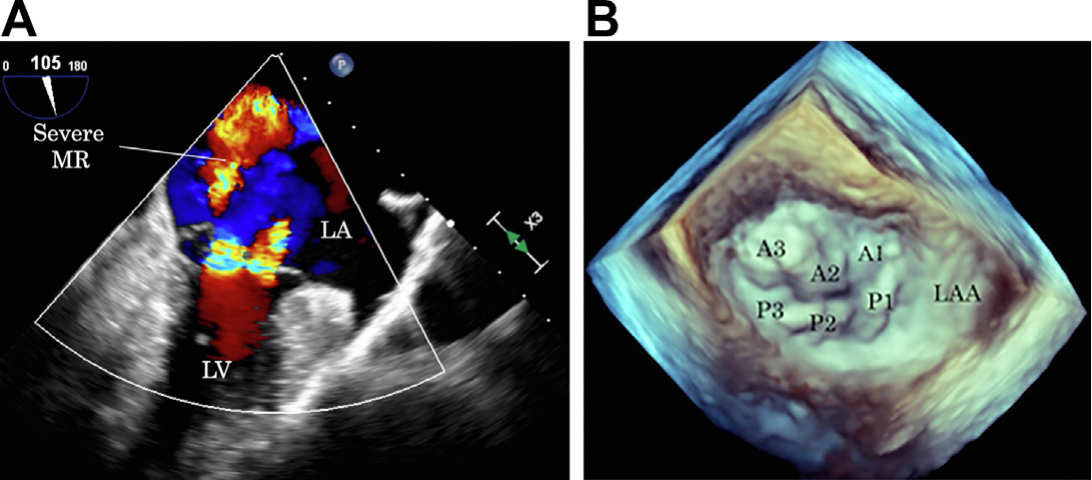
(A) Preoperative transesophageal echocardiography with color Doppler (midesophageal mitral commissural view) shows severe mitral regurgitation (MR) due to anterior leaflet prolapse. (B) Three-dimensional view of the mitral valve in dextrocardia as viewed intraoperatively by the surgeon standing on the left side of the patient. (LA, left atrium; LAA, left atrial appendage; LV, left ventricle; A1, the anterior segment of the anterior leaflet; A2, the middle segment of the anterior leaflet; A3, posterior segment of the anterior leaflet; P1, the anterior scallop of the posterior leaflet; P2, the middle scallop of the posterior leaflet; P3, the posterior scallop of the posterior leaflet.)
